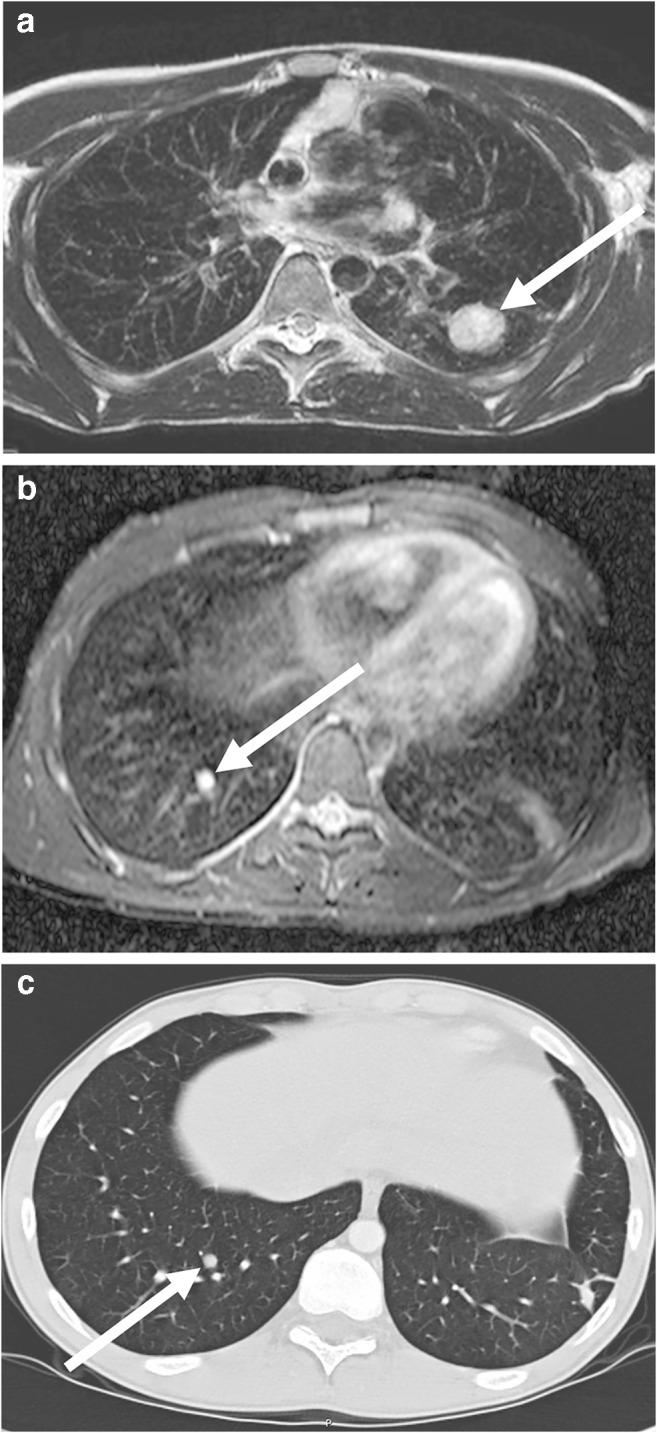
Axial lung MR in an 11-year-old boy with Ewing sarcoma and lung metastases (MR parameters in Table 2). a T2-weighted turbo spin-echo (TSE) image shows a 15-mm metastasis (*arrow*) in left upper lobe. b Fat-suppressed T2-weighted TSE image shows a 3-mm metastasis (*arrow*) in the right lower lobe. At MRI, metastases are just as easy to detect as they are at CT — they usually show a slightly brighter signal than that of vessels. This is especially true in heavily T2-weighted fat-suppressed sequences. c For comparison, axial CT image shows the same 3-mm metastasis (*arrow*) as the MR image in (b)
